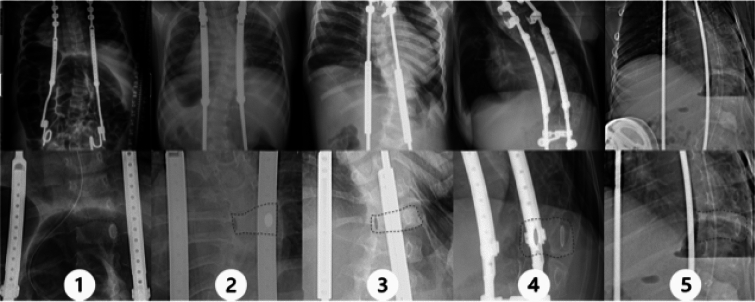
Radiographic appearance of example patients of grades 1–5 based on the location of the apical vertebra relative to the convex-sided spinal rod. The convex rod is lateral to the convex-sided pedicle for grade 1, overlaps the convex-sided pedicle for grade 2, lies between the pedicles for grade 3, overlaps the concave-sided pedicle for grade 4, and is medial to the concave-sided pedicle for grade 5
